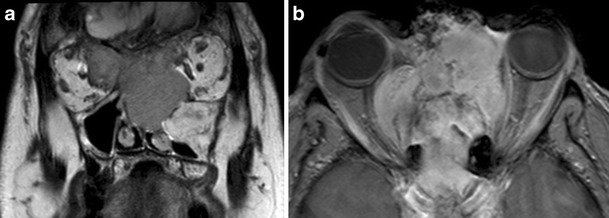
a) Coronal T2-weighted and b) axial post-contrast T1 fat-saturated MR images. The patient from Fig. 3 subsequently represented two years later with difficulty opening his right eye and changes in visual acuity. MRI demonstrated an enhancing mass lesion centered on the ethmoid sinuses, naso ethmoid complex and cribiform plate, which invades both orbits. There is invasion of the intraconal space of the right orbit, displacement of the right optic nerve and inferior lateral displacement of the right globe. The lesion was proven on biopsy to be an ossifying fibroma, although it behaved atypically in an invasive manner
